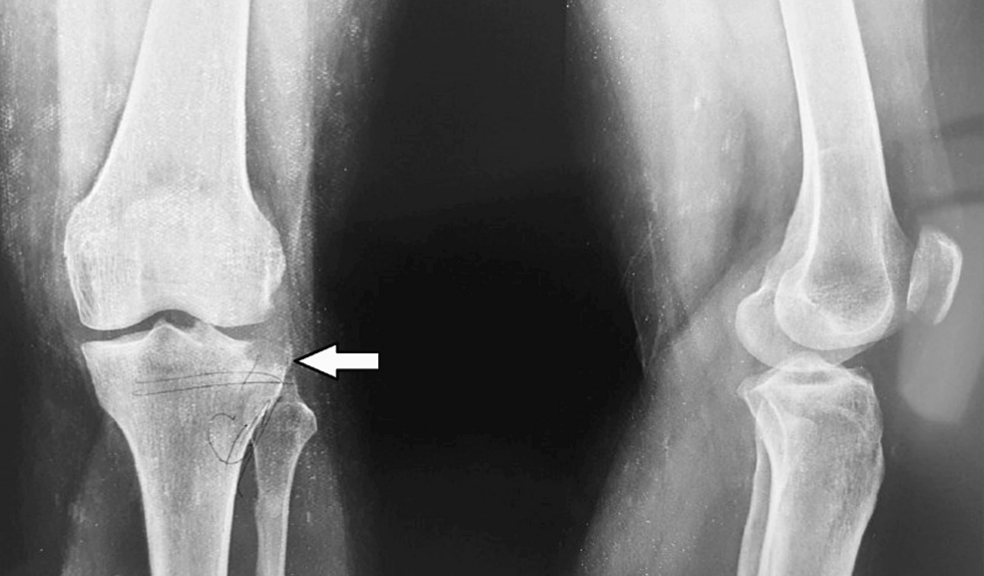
Preoperative X-ray of left knee (anteroposterior and lateral view) showing depressed intraarticular lateral tibial plateau fracture (white arrow)
